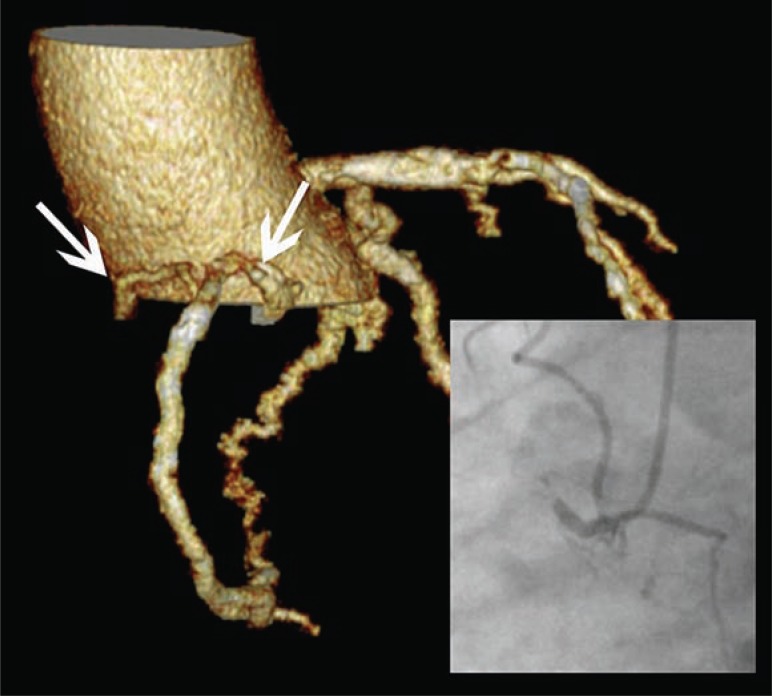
Use of co-registered cardiac CT to aid CTO PCI. Marked ambiguity existed in the proximal occluded segment with regard to course
